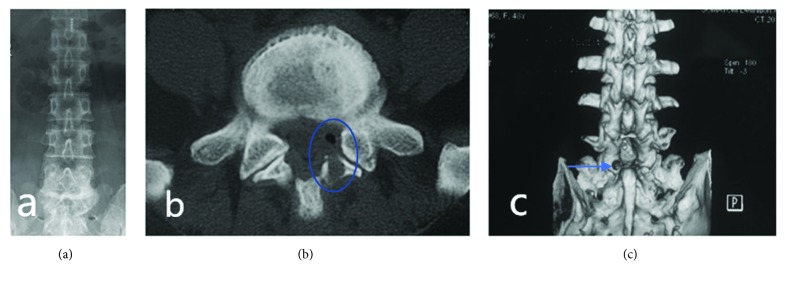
(a) Postoperative lumbar anteroposterior. (b) Posterior lumbar CT showing full left septal decompression. (c) Lumbar vertebra CT + 3D reconstruction showing the articular process sawing window decompression zone (arrows).
